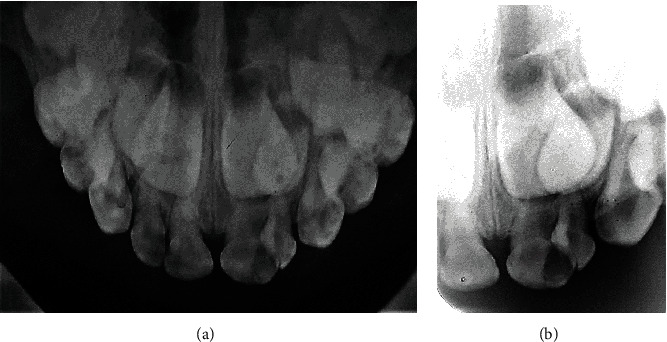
Radiographic examination showing the morphology type: tooth 61 with a single root and a large central root canal. Periapical radiograph (a). Maxillary occlusal radiograph (b).
